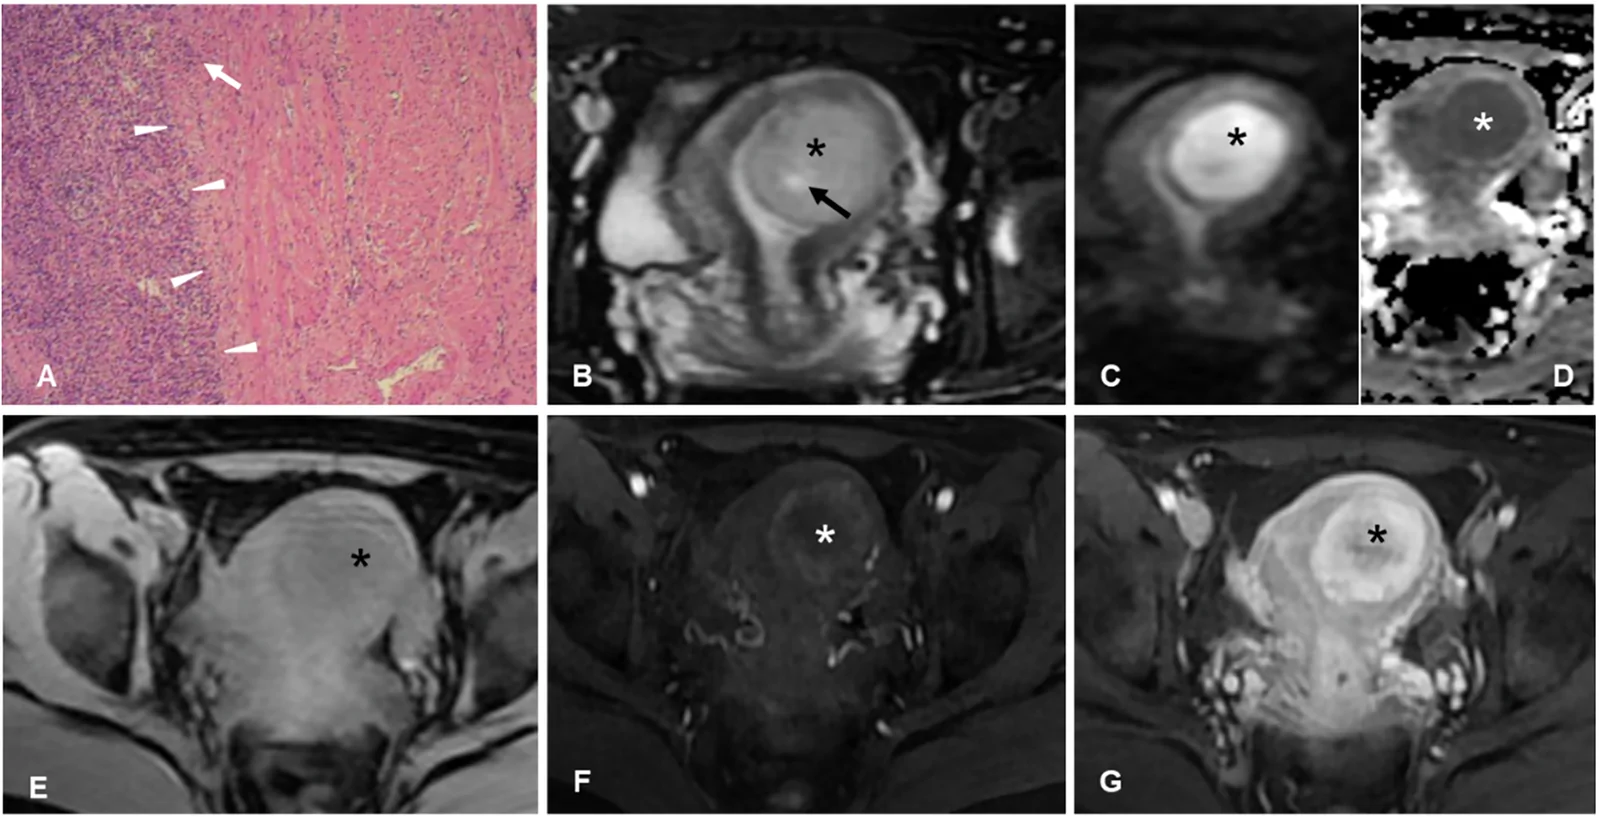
Pathological and magnetic resonance images of an ESN in a 42-year-old woman. Pathological image (A) demonstrating the ESN sharply demarcated from the surrounding myometrium. Axial T2WI (B) shows that the tumor (asterisk) has high T2WI signal intensity and an absence of a speckled appearance, along with small cystic changes within the tumor (arrow). DWI (C) and an ADC map (D) demonstrate restricted diffusion within the tumor. Axial T1-WI (E) depicts the tumor as slightly hypointense. Axial contrast-enhanced T1-WI (F, G) demonstrates marked progressive enhancement of the tumor
